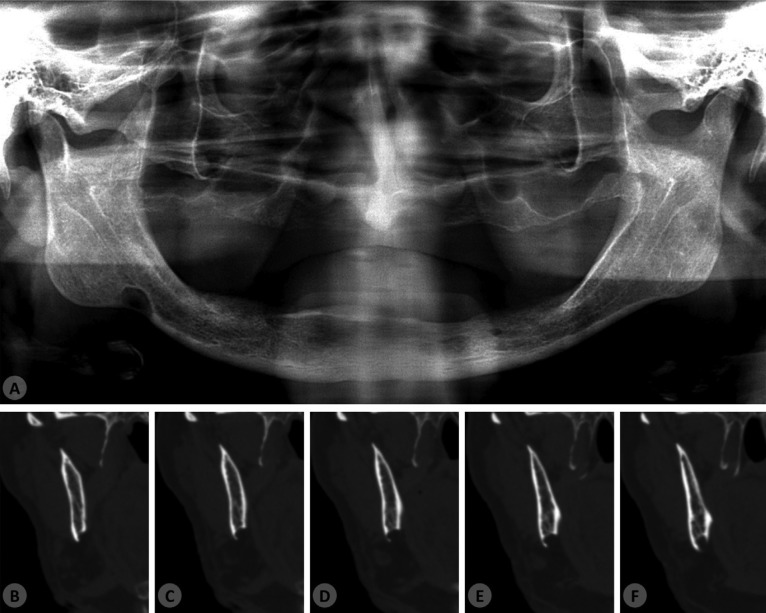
(A) Panoramic radiography showing a well-defined unilocular radiolucent area in the right posterior portion of the mandible near to the angle and in close contact to the inferior border. (B-F) Coronal CT views demonstrating the bone concavity affecting the lingual and inferior aspects of the mandible.
